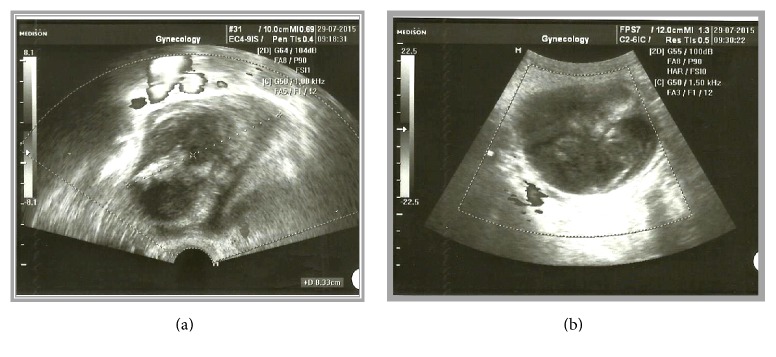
Transvaginal ultrasound showed a cystic formation sized 83 × 65 × 64 mm in the right adnexal area with signs of necrosis.
